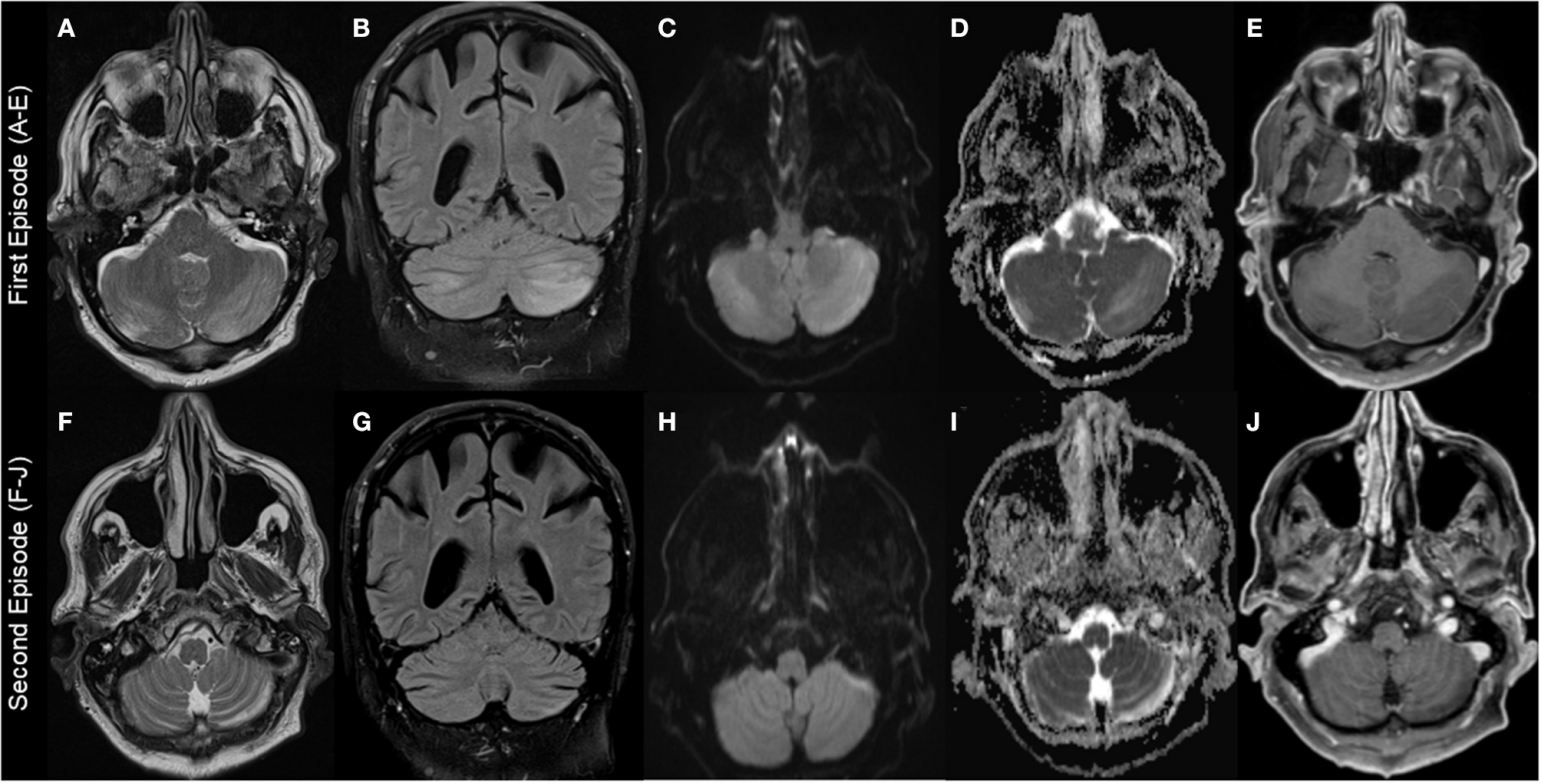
Brain MRI findings. First episode: Images (A–E); second episodes (F–J) (resembling residual findings on further follow up MRIs): (A+F): T2-weighted-images (T2W); (B+G): fluid-attenuated inversion recovery (FLAIR) images; (C+H): diffusion-weighted images (DWI); (D+I): apparent diffusion coefficient (ADC) images; (E+J): T1-weighted images (T1W) with gadolinium (Gd). Images show left-dominant cerebellar hyperintense lesions on T2W, FLAIR, and ADC images that are hypointense without Gd enhancement T1W images. DWI images at first episode showed hyperintense lesion and were normal at second episode.
